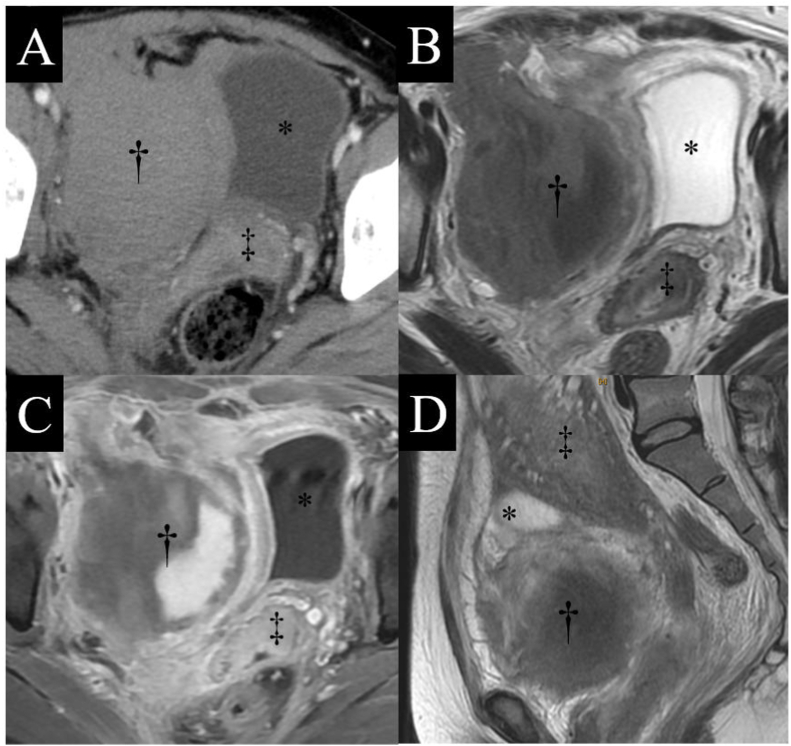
Preoperative images. Panels A–C are axial images of the positioning of the retroperitoneal hematoma and pelvic organs, and D is a sagittal image of the same. A) Contrast-enhanced computed tomography revealed no extravasation into the hematoma. B) and D) T2-weighted MRI revealed a hematoma on the right side of the pelvis, near the upper vaginal wall. C) Contrast-enhanced, T1-weighted MRI revealed a cystic hematoma with a contrast effect. The hematoma, uterus, and bladder are indicated as †, ‡, and *, respectively. MRI: magnetic resonance imaging.
